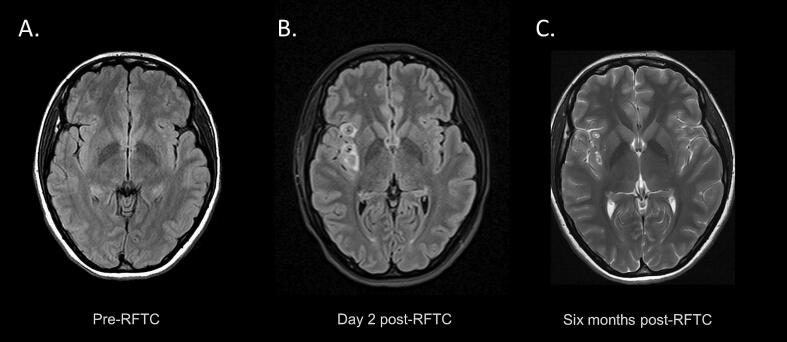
MRI changes following radiofrequency thermocoagulation. Axial T2/FLAIR-weighted MRI sequences showing insular structures before radiofrequency thermocoagulation (RFTC) (A), T2 signal hyperintensities surrounding ablation sites two days following RFTC (B), and residual changes six months after RFTC (C).
